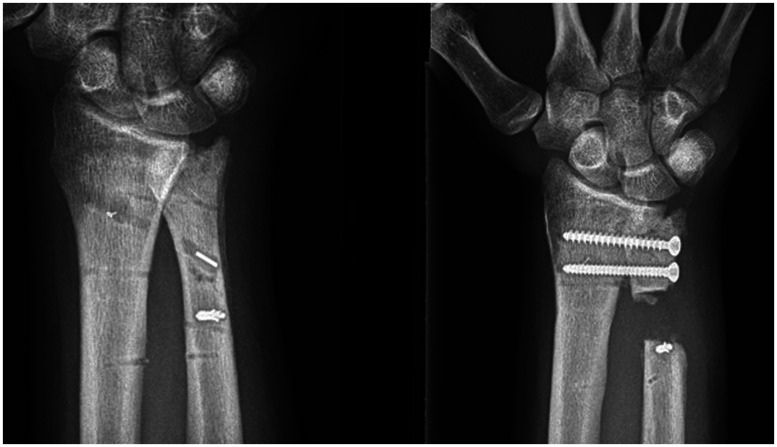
(Left) Preoperative and (right) postoperative radiographs of a patient in this study. The postoperative image shows a clear view of the screws and pseudoarthrosis.
